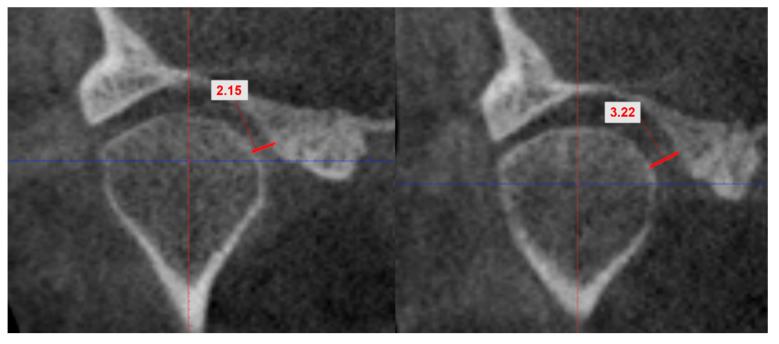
CBCT coronal section. The measurements of the minimal length of the medial joint space.
